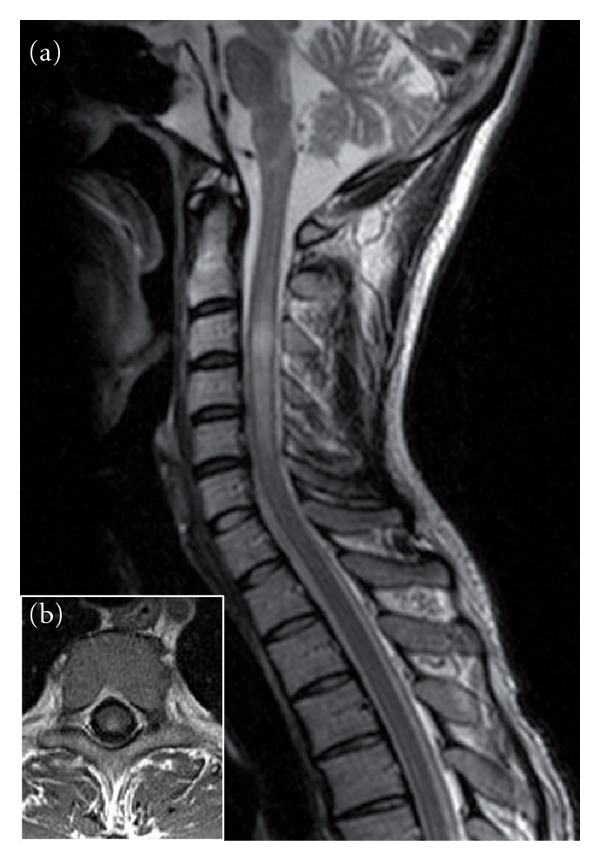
Sagittal (a) and axial (b) T2-weighted MRI sequences disclose cervical spinal cord hyperintensity in a patient with HCV-related myelitis.
